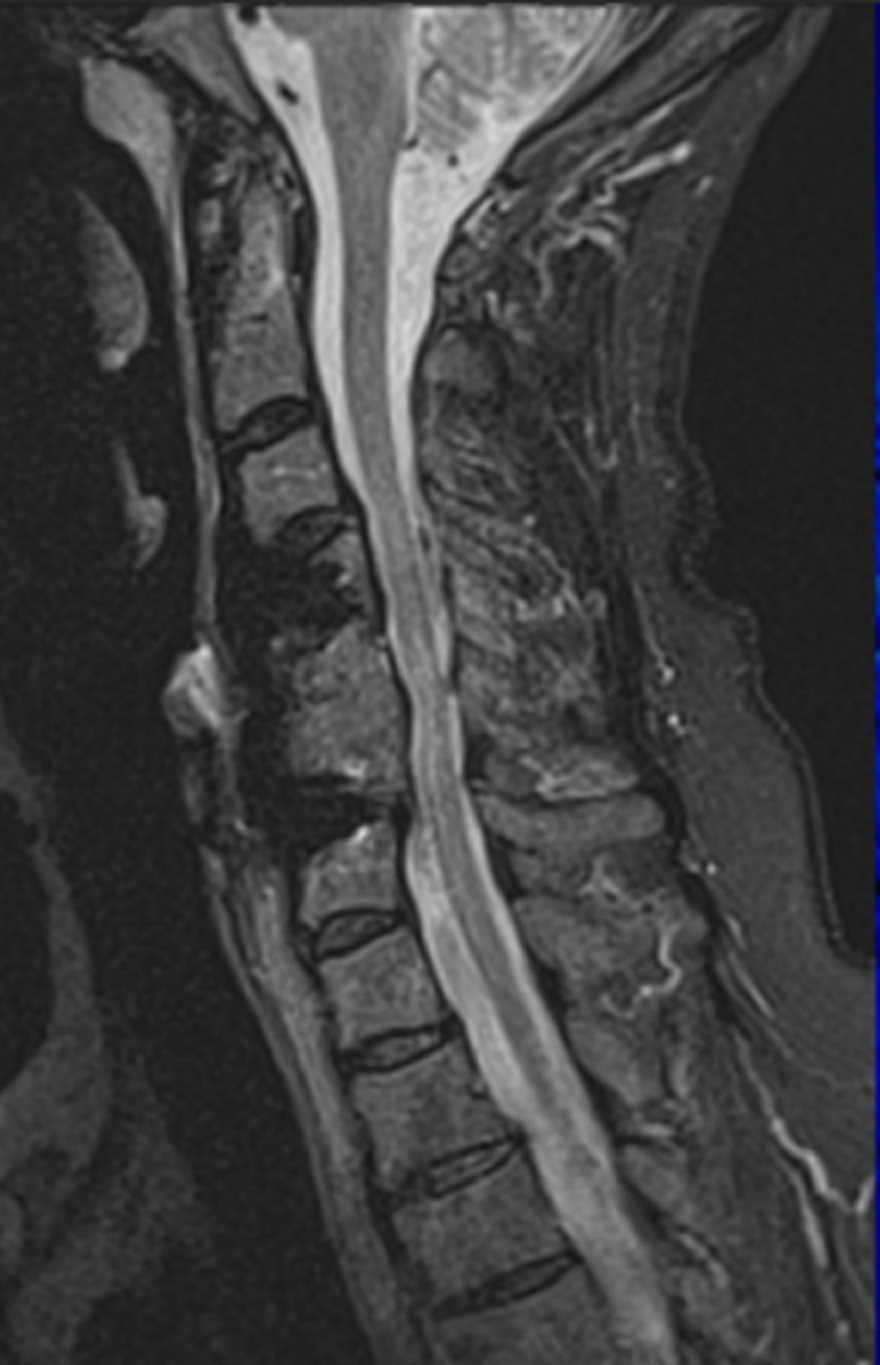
(Case 2) Preoperative sagittal MRI of the cervical spine
